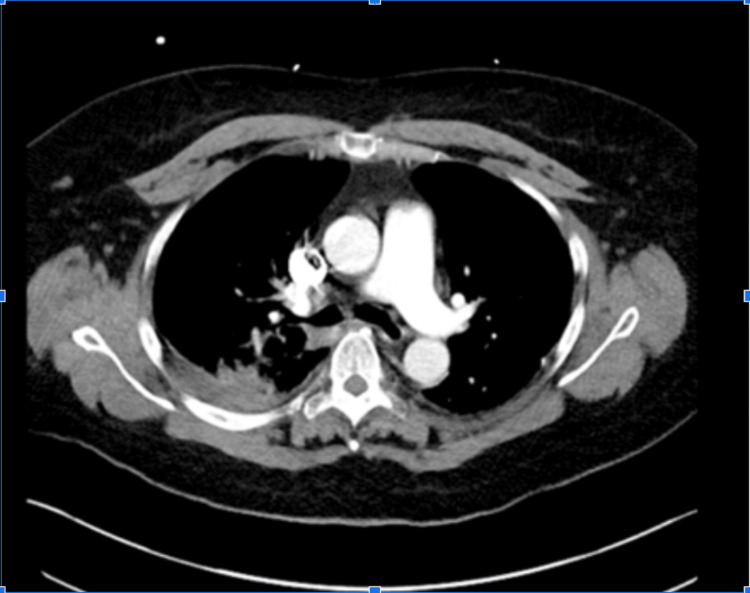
Chest CT performed on Day 1
